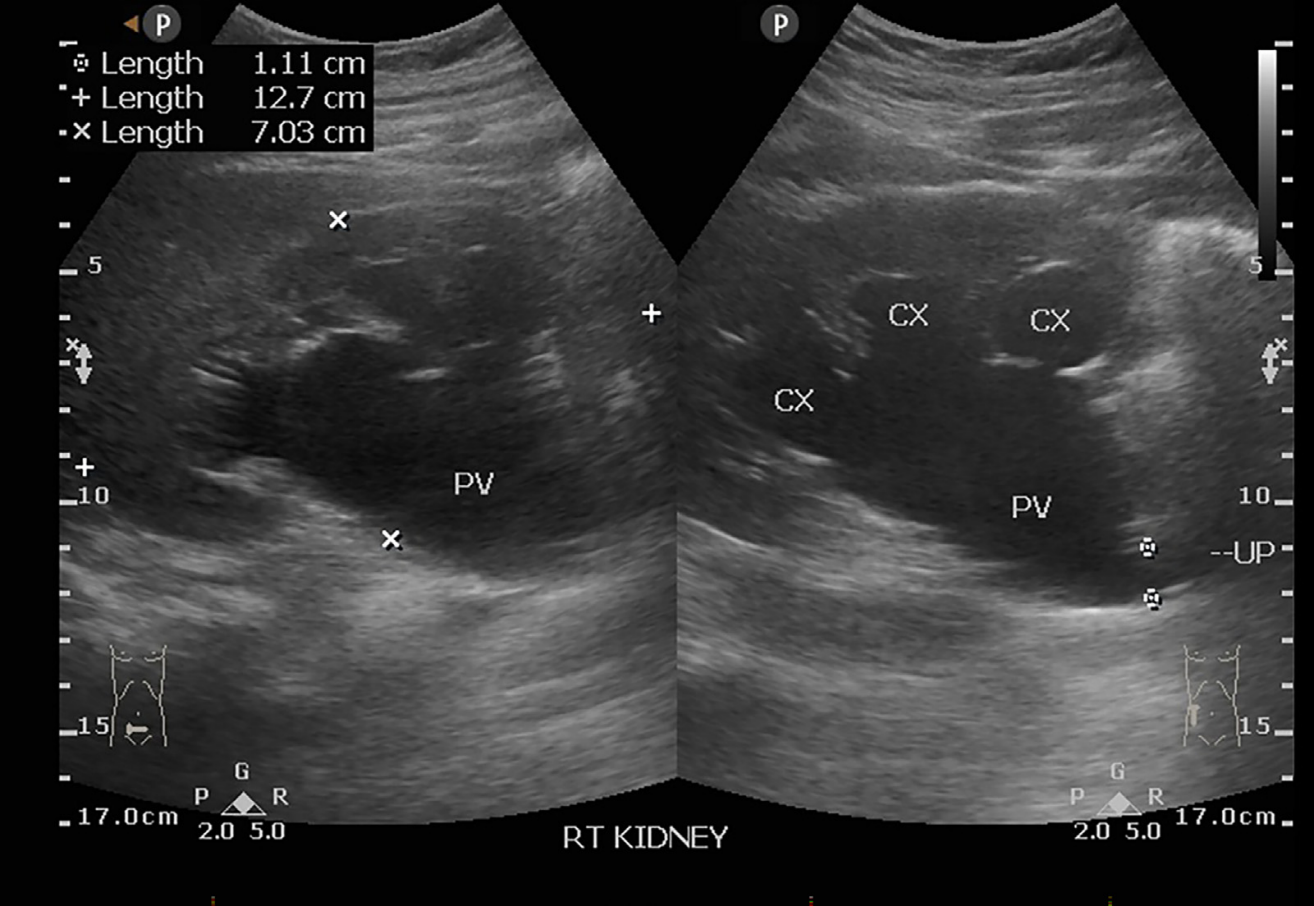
Longitudinal ultrasound of the right kidney demonstrated pelvicalyceal dilatation and a dilated proximal ureter. CX, calyx; PV, Pelvis.
